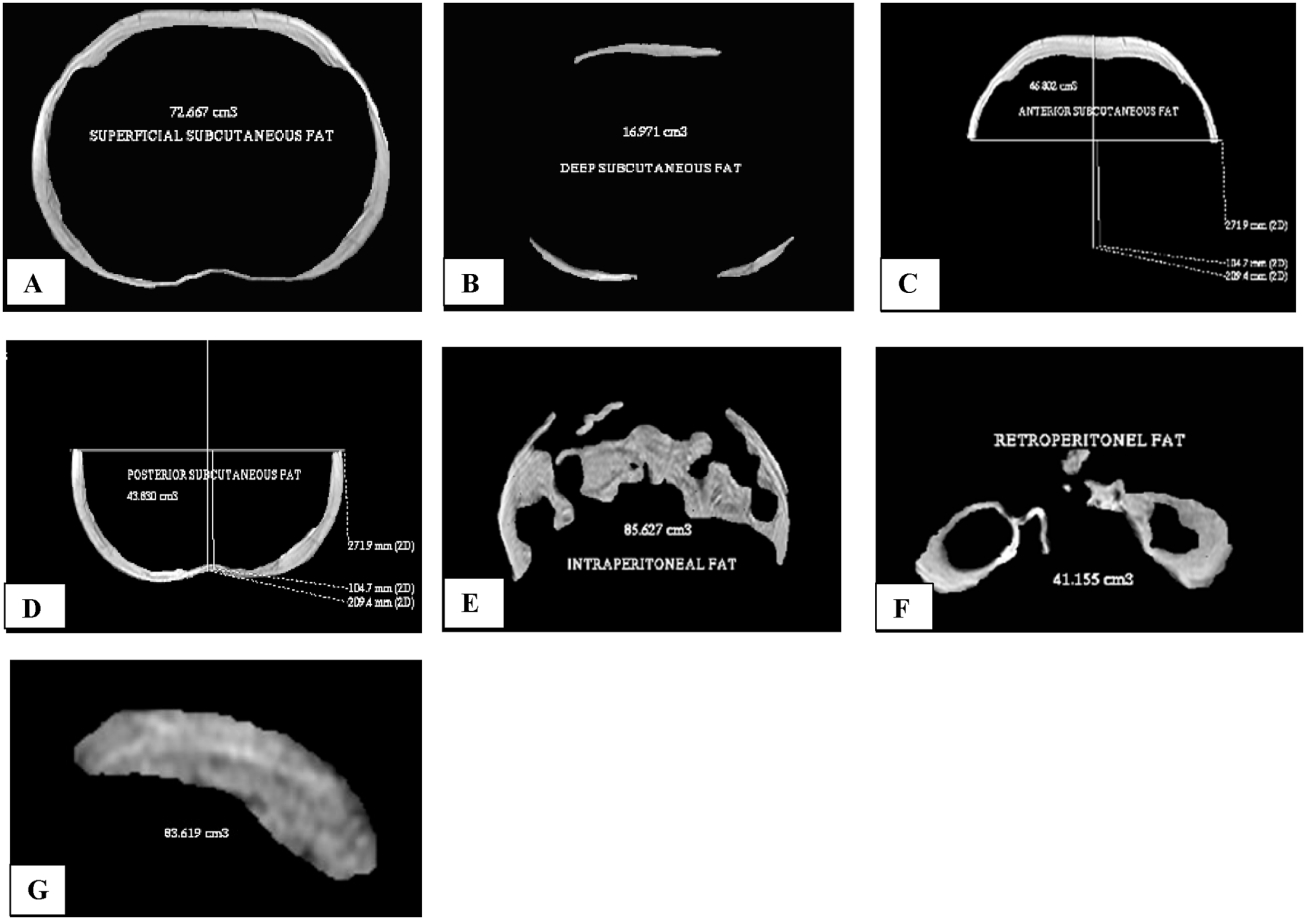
Abdominal fat depots and pancreatic volume in a 33 year old non-obese patient with type 2 diabetes, quantified from image obtained using MRI (1.5 Tesla) at L2/L3 region of lumbar vertebrae (A): Superficial abdominal subcutaneous fat, (B): Deep abdominal subcutaneous fat, (C): Anterior abdominal subcutaneous fat, (D): Posterior abdominal subcutaneous fat, (E): Intra-peritoneal fat, (F): Retroperitoneal fat & (G): Pancreatic volume.
